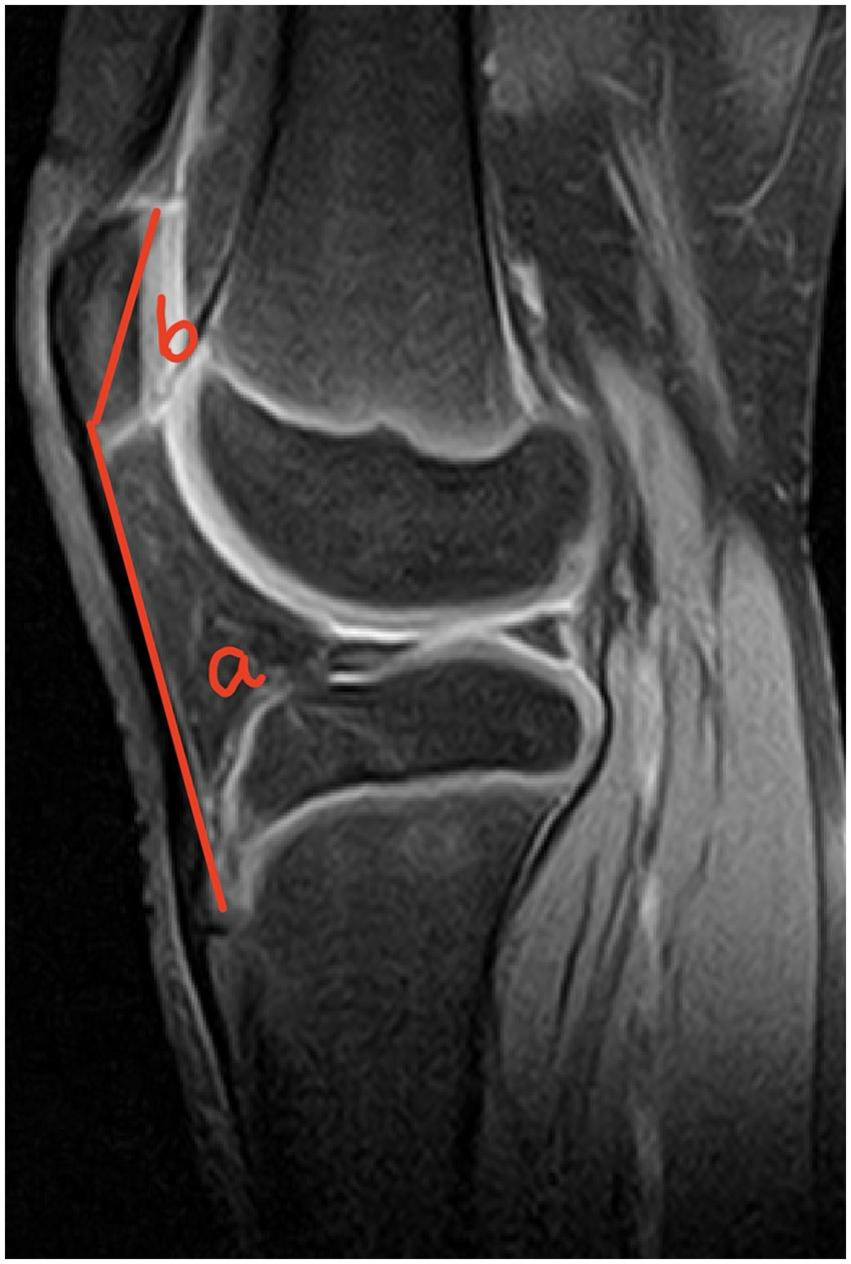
Sagittal MRI scan of the knee joint showing key anatomical measurements (a, b) for ISI calculation.
